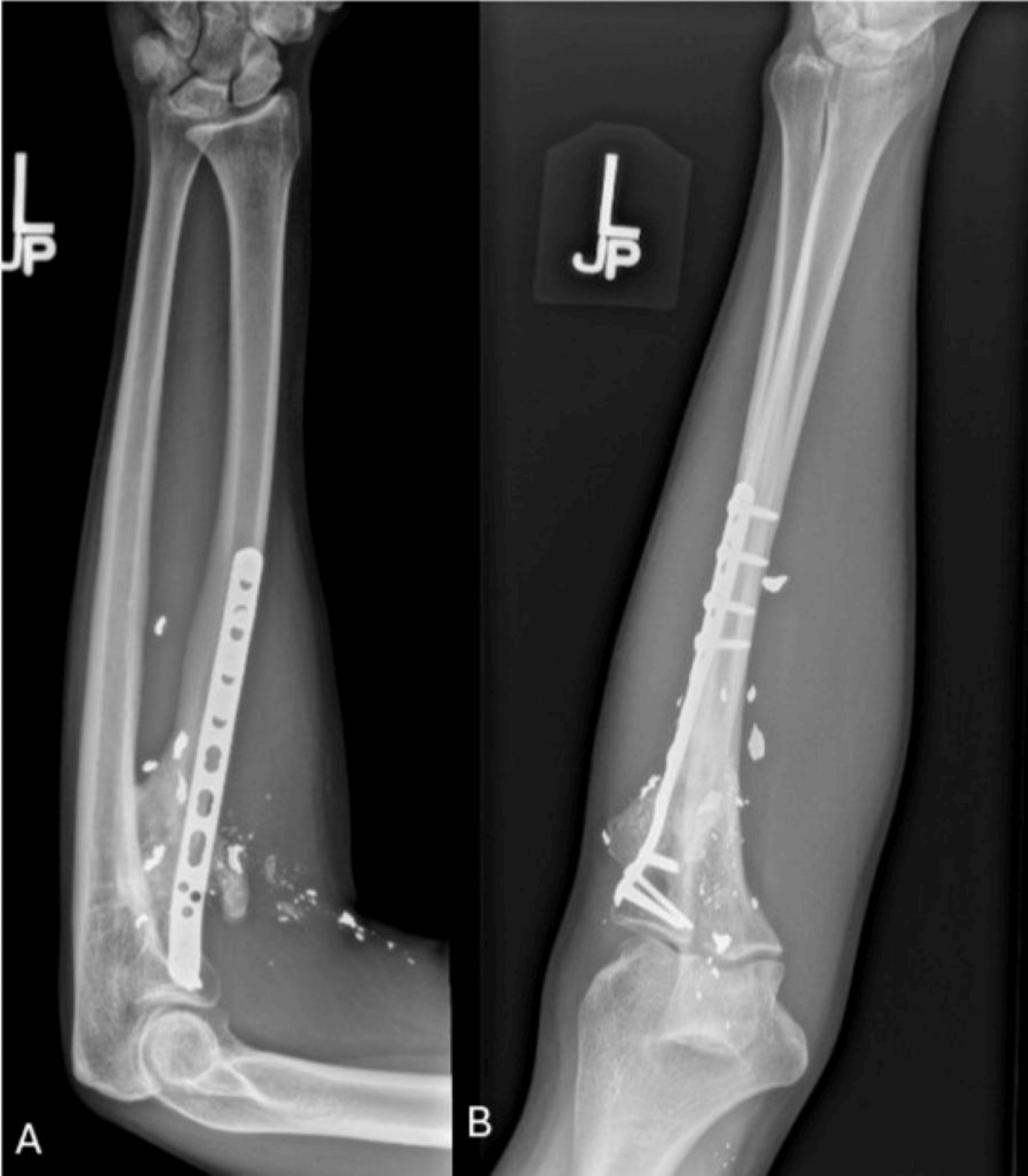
Final left elbow radiographs Anteroposterior (A) and lateral (B) radiographs demonstrating a healed proximal radial shaft fracture at 24 months postop fixed with a right-sided posterolateral distal fibula plate.
